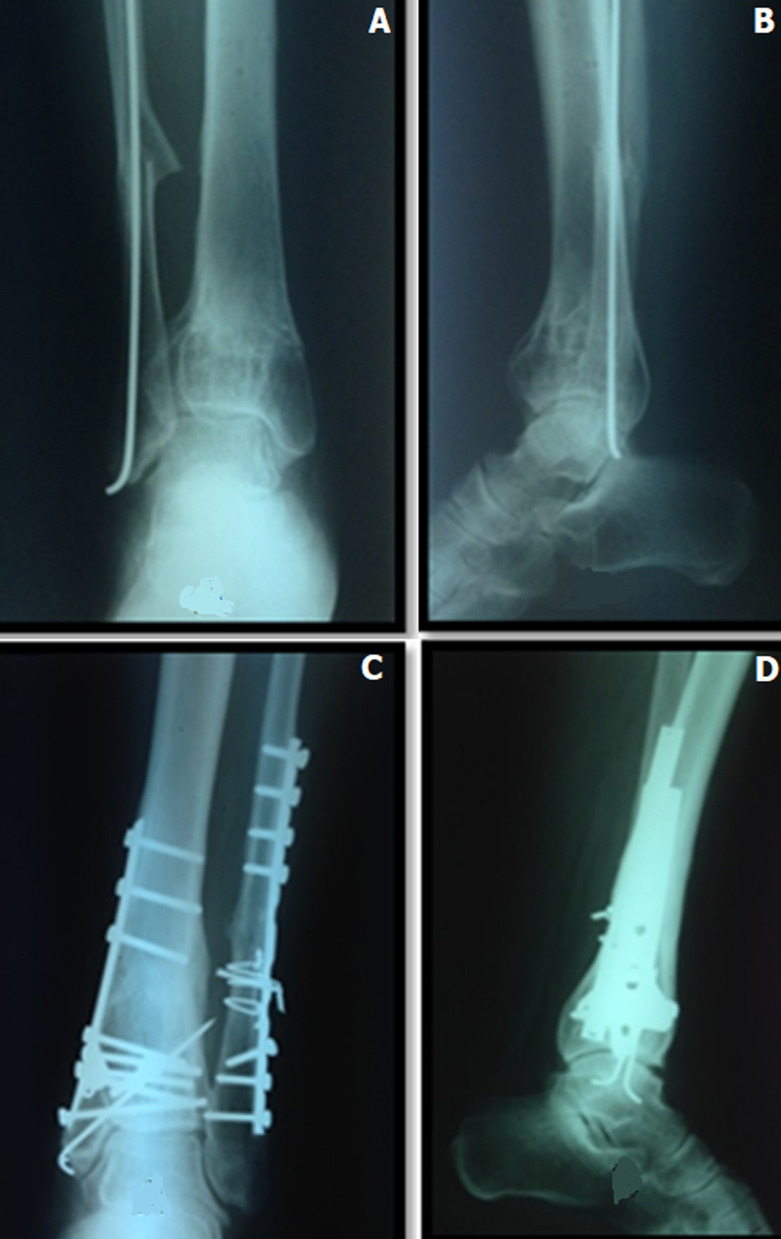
radiographie de la cheville de face (A) et de profil (B) montrant une consolidation de la fracture après fixation externe (C) et synthèse interne (D)
